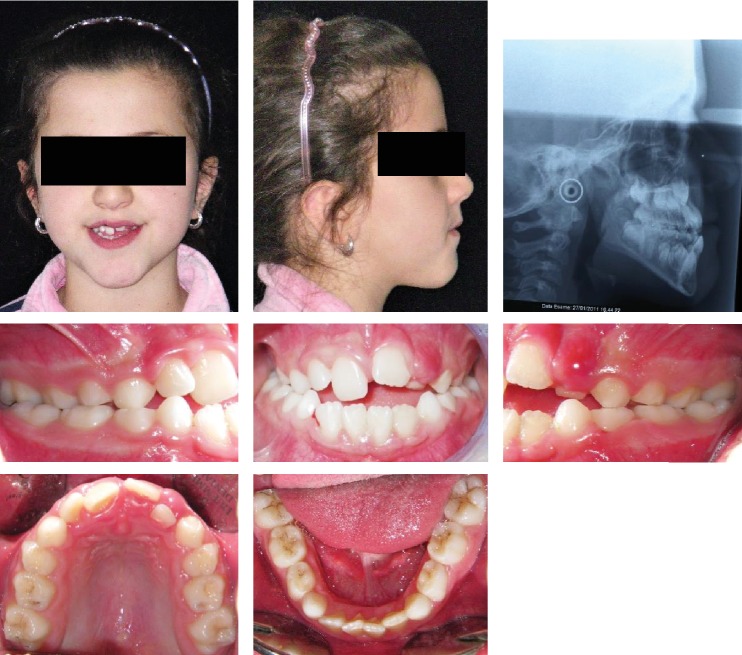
Case no. 2 facial photos, intraoral photos, panoramic radiograph, and lateral cephalogram at *T*_0_ (pretreatment).
